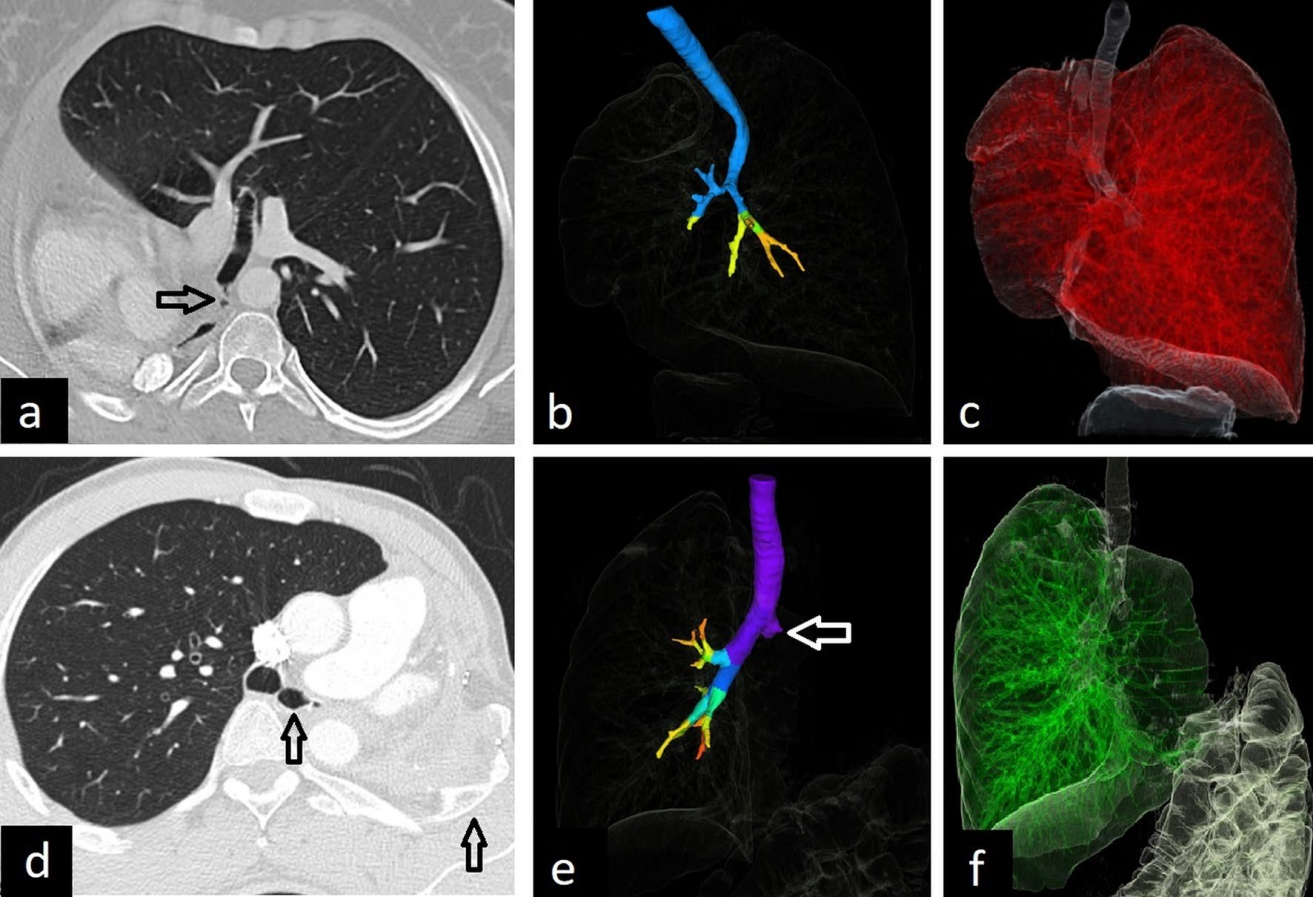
CT images of pulmonary aplasia and pneumonectomy. Rudimentary blind-ending bronchus can be seen on axial CT image in a 27-year-old asymptomatic female with pulmonary aplasia and helps in the differentiation from pulmonary agenesis (a) (arrow). 3D reformatted images (b, c) show the only left airways and compensatory hyperplasia of the left lung into the right side. The left main bronchial stump is observed on axial CT and 3D reformatted images in a 33-year-old patient with right pneumonectomy (d–f) (arrows). Rib deformities and pleural fluid due to pneumonectomy are depicted on axial CT image (d) (arrow)
